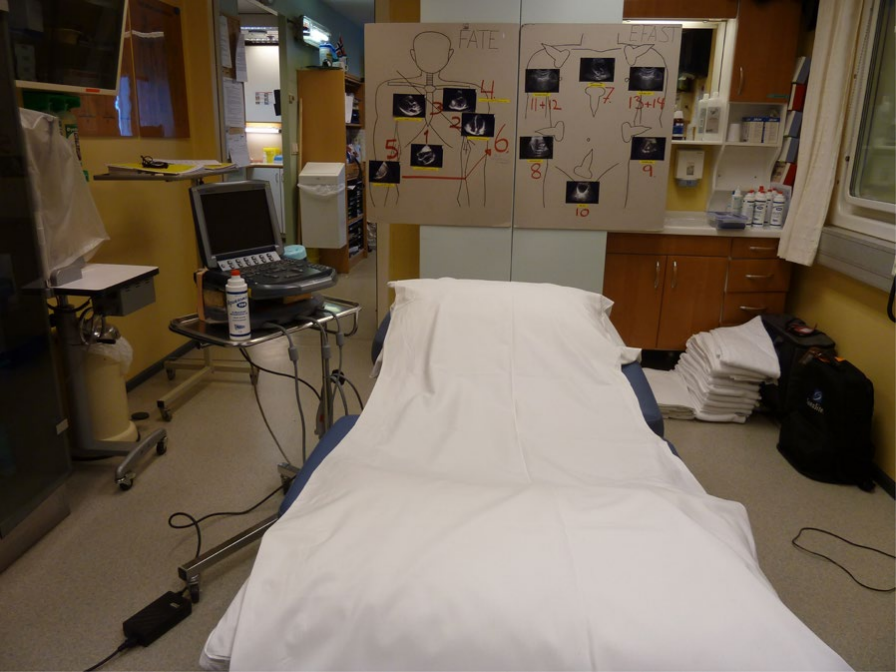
Scanning positions. Offshore hospital bed with M-Turbo ultrasound machine and wall-mounted scanning cards for Focused Assessed Transthoracic Echo (FATE) and extended Focused Assessment with Sonography for Trauma (e-FAST). Ultrasound video clips and images were recorded from all 14 positions. Photo: Nils Petter Oveland
